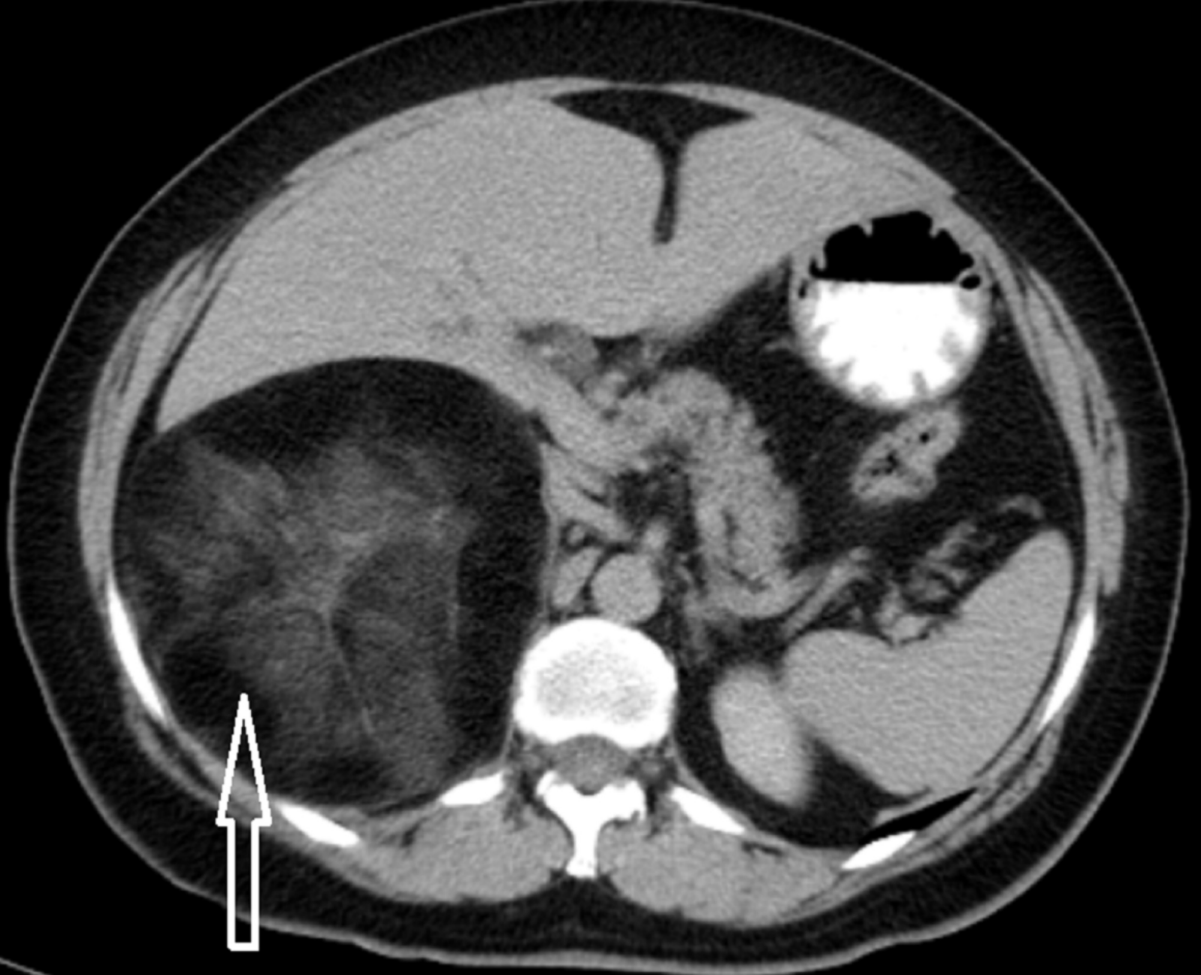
Preoperative abdominal CT scan demonstrating a 12.9 × 11.7 cm in size, encapsulated, hypodense, solid, right retroperitoneal mass (arrow), containing fat (mainly), interspersed soft-tissue attenuation components and a few septa. The tumor displaced liver’s right lobe anteriorly.
